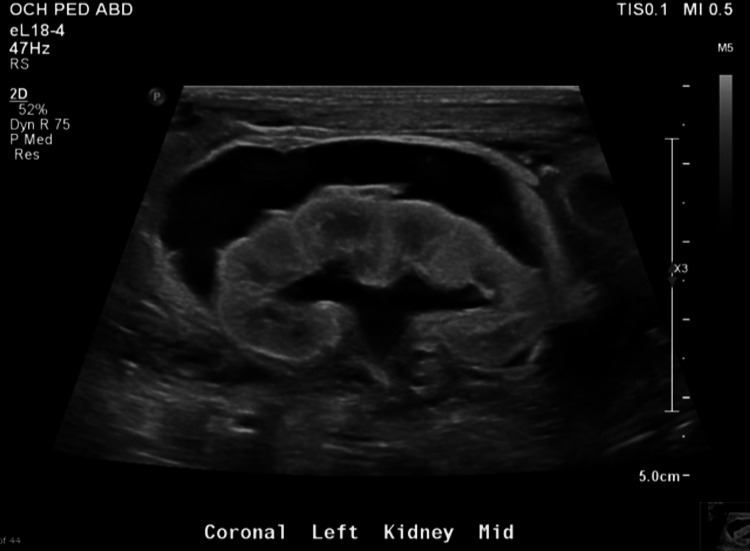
Ultrasound of left kidney on DOL1 demonstrating mild hydronephrosis, normal-appearing fetal renal parenchyma, and persistent left subcapsular fluid collection (initially seen on prenatal ultrasound). DOL: Day of life
